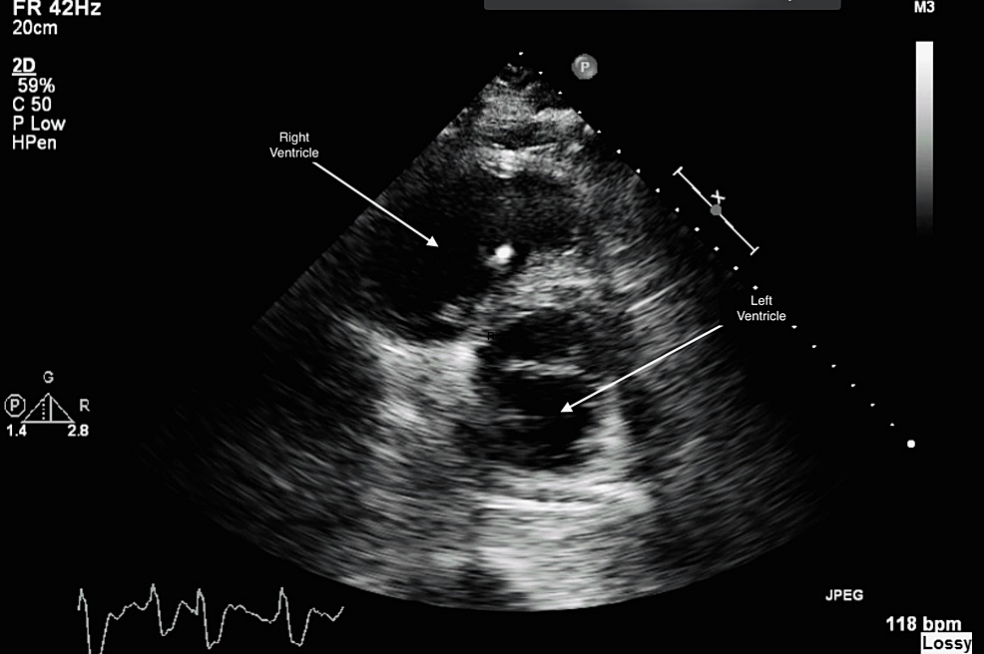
TTE parasternal short-axis view at mid-left ventricular cavity level demonstrating reduced biventricular function TTE: transthoracic echocardiography
